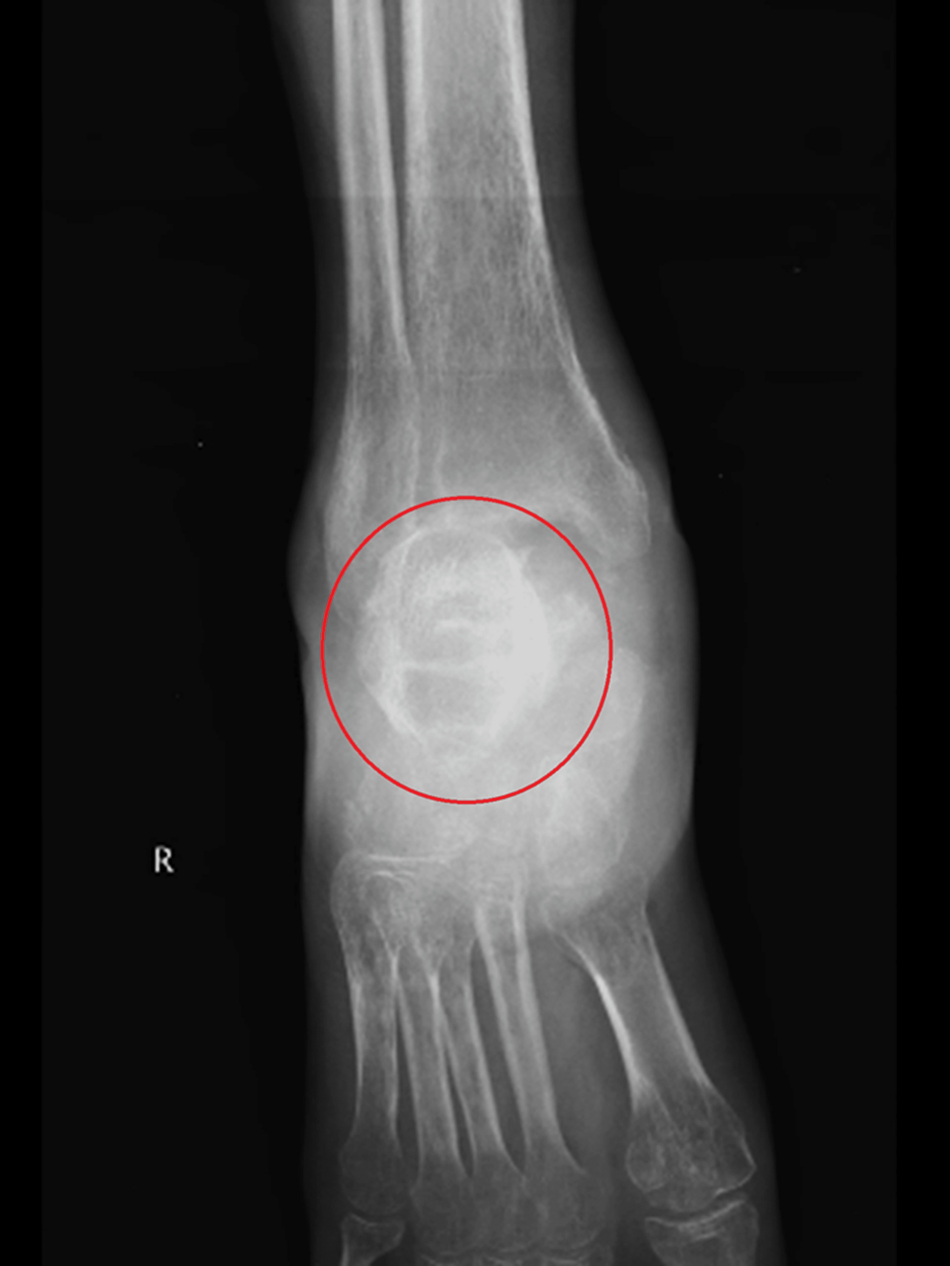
X-ray of the right ankle joint from the frontal view showing the arthritic changes of the talus bone.
